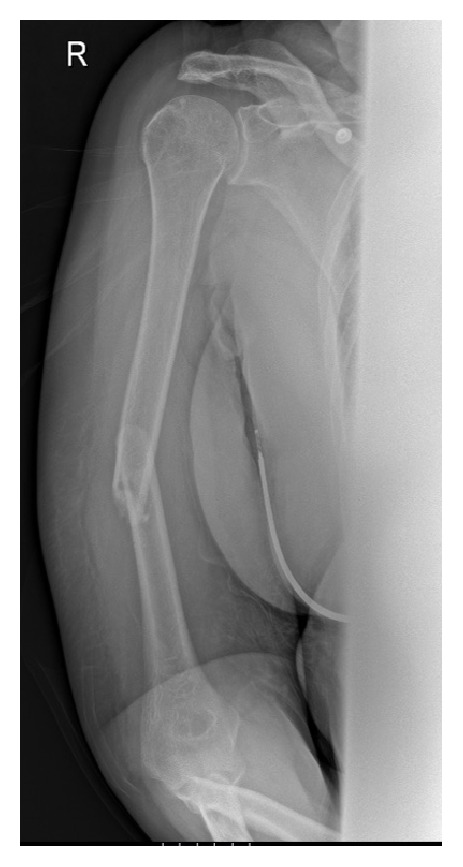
Initial AP radiograph showing a mid-diaphyseal humeral fracture with mild angulation.
